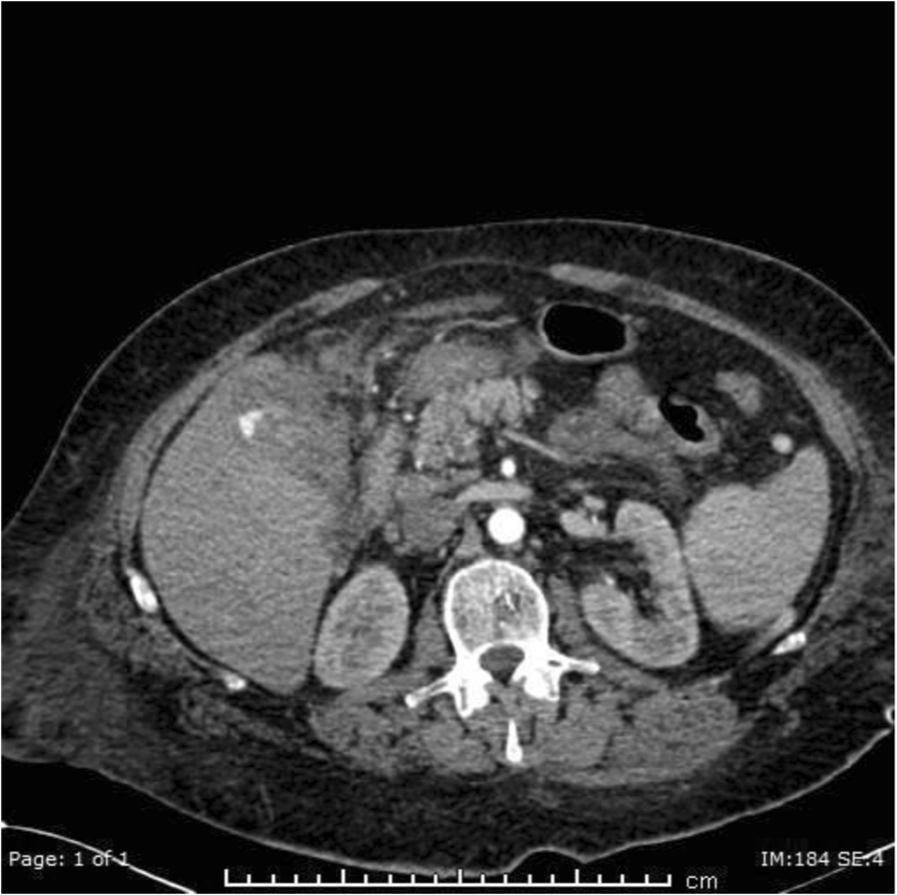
Arterial phase CT. Contrast blush within the gallbladder
